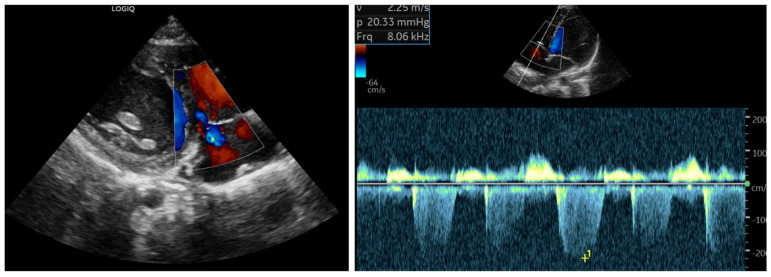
Follow-up echocardiography (7 days post−treatment): complete resolution of the midsystolic notching and normalization of the estimated systolic pulmonary artery pressure (<25 mmHg) following one week of intravenous vitamin C and multivitamin supplementation.
